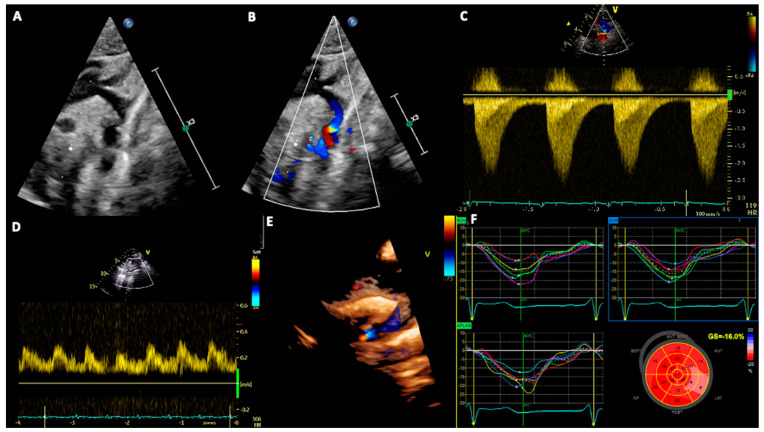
Panel (A,B): Coarctation of the aorta as seen by 2D and color Doppler. Panel (C): CW Doppler flow pattern, showing the typical saw-tooth appearance, with antegrade flow extending into diastole. Panel (D): Typical flow pattern in the abdominal aorta. Panel (E): Three-dimensional image of CoA. Panel (F): GLS values, which are typically reduced in basal segments.
